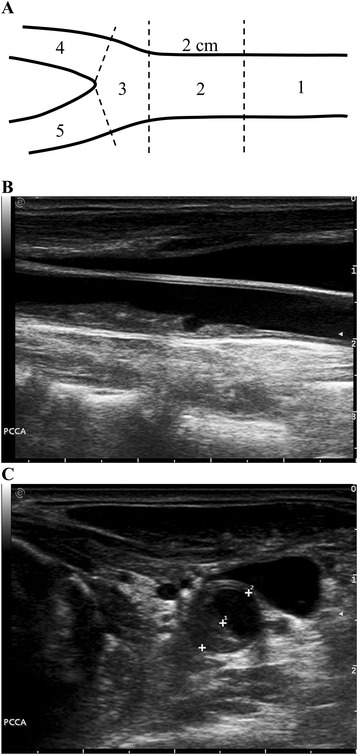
Assessment of carotid plaque score. A). Five segments of the extra-cranial carotid artery: 1. proximal common carotid artery (≥2 cm proximal to carotid bifurcation); 2. distal common carotid artery (<2 cm proximal to carotid bifurcation); 3. carotid bulb and bifurcation; 4. internal carotid artery; and 5. external carotid artery. B). A longitudinal gray-scale image of a carotid plaque in the distal common carotid artery. C). A transverse gray-scale ultrasound image of the carotid plaque in B. The degree of the carotid stenosis is expressed as the percentage reduction of lumen diameter (1-D1/D2 = 41.3%). Carotid plaque score is the summation of the degree of carotid stenosis of the five segments in both carotid arteries.
